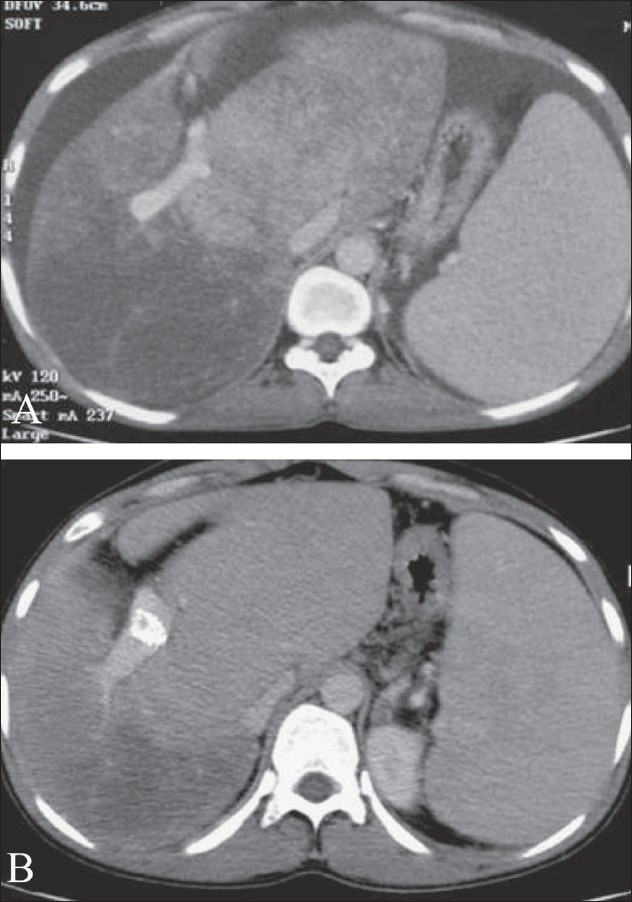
Contrast-enhanced CT scan of a patient with Budd-Chiari syndrome, before TIPS (A) and after TIPS (B), showing improvement in enhancement pattern of the liver parenchyma and regression of ascites following TIPS
